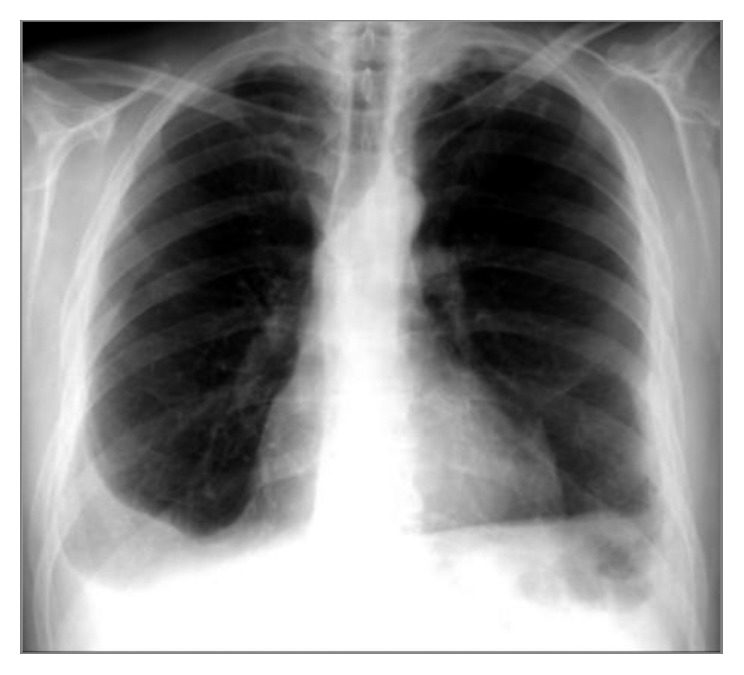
Chest radiography showing right-sided pleural effusion and left diaphragmatic retraction.
